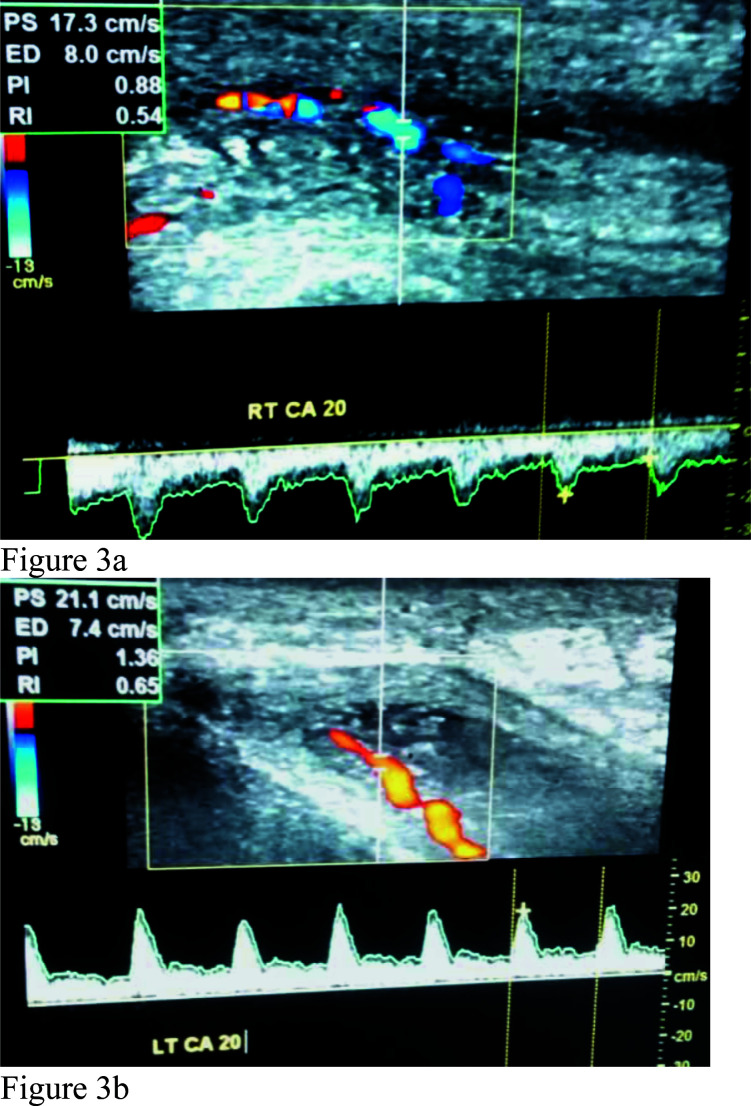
Triplex Doppler waveforms of the right and left cavernosal arteries at 20 minutes showing multiple areas of narrowing and dilatations, giving beaded appearances. Note the peak systolic velocities of 17.3cm/s and 21.1cm/s on the right and left side respectively.
